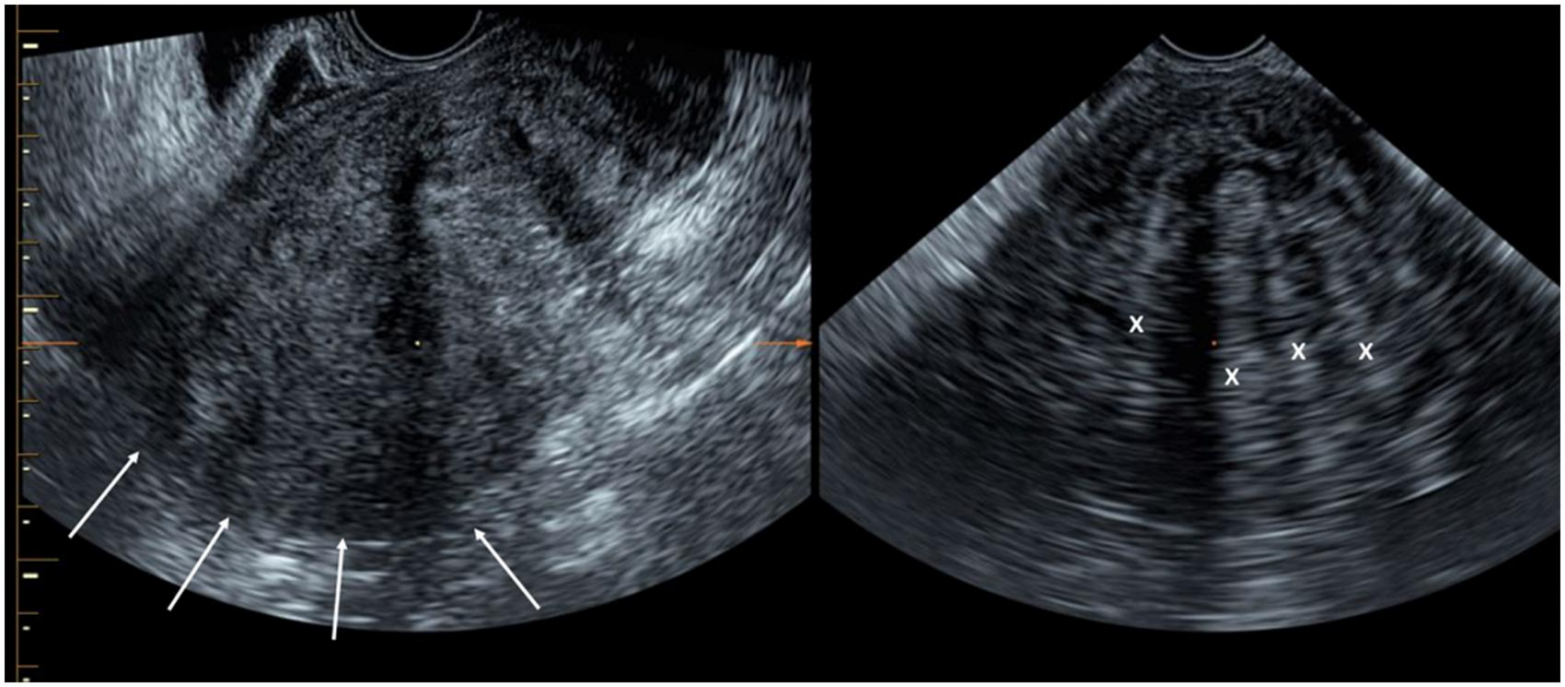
Long and transverse ultrasound images of a 43-year-old female with known Müllerian Duct anomaly (not characterised on these images) and endometriosis showing anteverted retroflexed uterus with a globular fundus (arrows), hyperechoic islands, heterogeneous myometrium with loss of clarity of the normal junctional zone and fan-shaped shadowing (x), which are suggestive of diffuse adenomyosis. It is important to note that the presence of hyperechoic islands is a direct ultrasound feature, while the remainder of the described sonographic findings represent the indirect features of adenomyosis as defined by the revised MUSA criteria.
